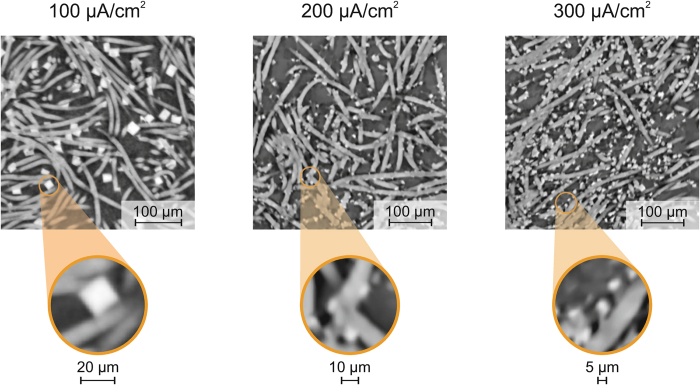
X-ray synchrotron tomography results: In-plane cross sections of selected areas showing the top (from O_2_ reservoir) of the cathodes analyzed for the current density indicated; discharge capacity 1.5 mAh; discharge product NaO_2_ (white color), fibers of the carbon cathode (gray color) and pore space (black color).
